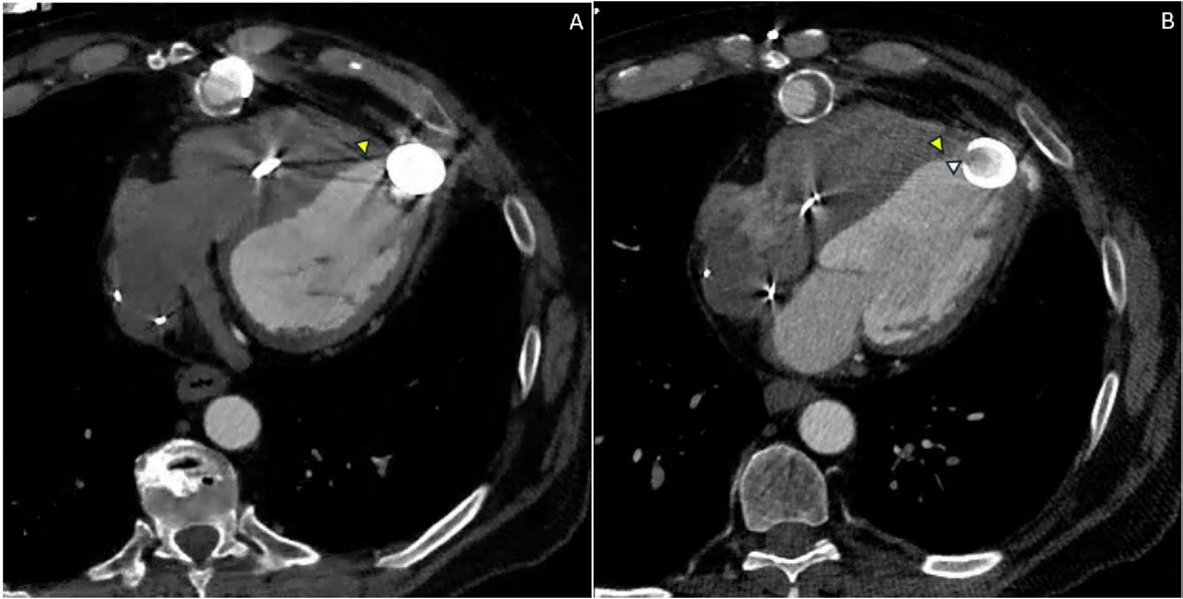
Axial multiphasic cardiac computed tomography angiography (CTA) demonstrating spatial orientation of the left ventricular assist device (LVAD) inflow cannula and phase-dependent septal apposition. A Axial diastolic phase cardiac CTA image demonstrates the LVAD inflow cannula (above the level of cannula orifice) directed toward the interventricular septum, with preserved but narrowed inflow channel as septal myocardium contacts the cannula orifice (arrowhead). B Axial diastolic phase at a more inferior slice level at the orifice of LVAD inflow cannula demonstrates progression to near-complete inflow obstruction as septal myocardium is drawn deeper into the cannula orifice (arrowhead). The inflow cannula orifice is indicated by the arrow, highlighting the dynamic and cyclical nature of the obstruction
